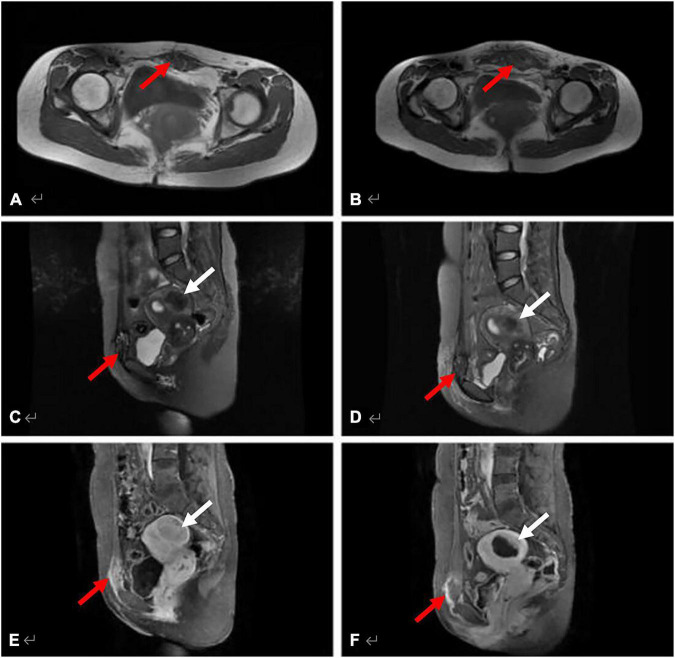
MRI images of AWE in a 42-year-old woman before and after HIFU treatment. The AWE lesion located in the ventral rectus abdominis, invading the rectus sheath. (A) Axial T1WI shows the lesion appeared as mixed signal (red arrow) pre-operatively. (B) Axial T1WI shows the same lesion appeared slightly hyperintense post-HIFU ablation (red arrow). (C,D) Sagittal T2WI with fat-suppression AWE lesion in the rectus abdominis shows high signal (red arrow), and the hysteromyoma in the posterior wall of the uterus appeared hypo-intense as compared with muscle (white arrow). (E) The AWE lesion (red arrow) and the hysteromyoma (white arrow) show evident enhancement and slight enhancement, respectively, on post-contrast T1WI. (F) Both the AWE lesion (red arrow) and the hysteromyoma (white arrow) clearly showed the non-enhancing areas of post-treatment.
